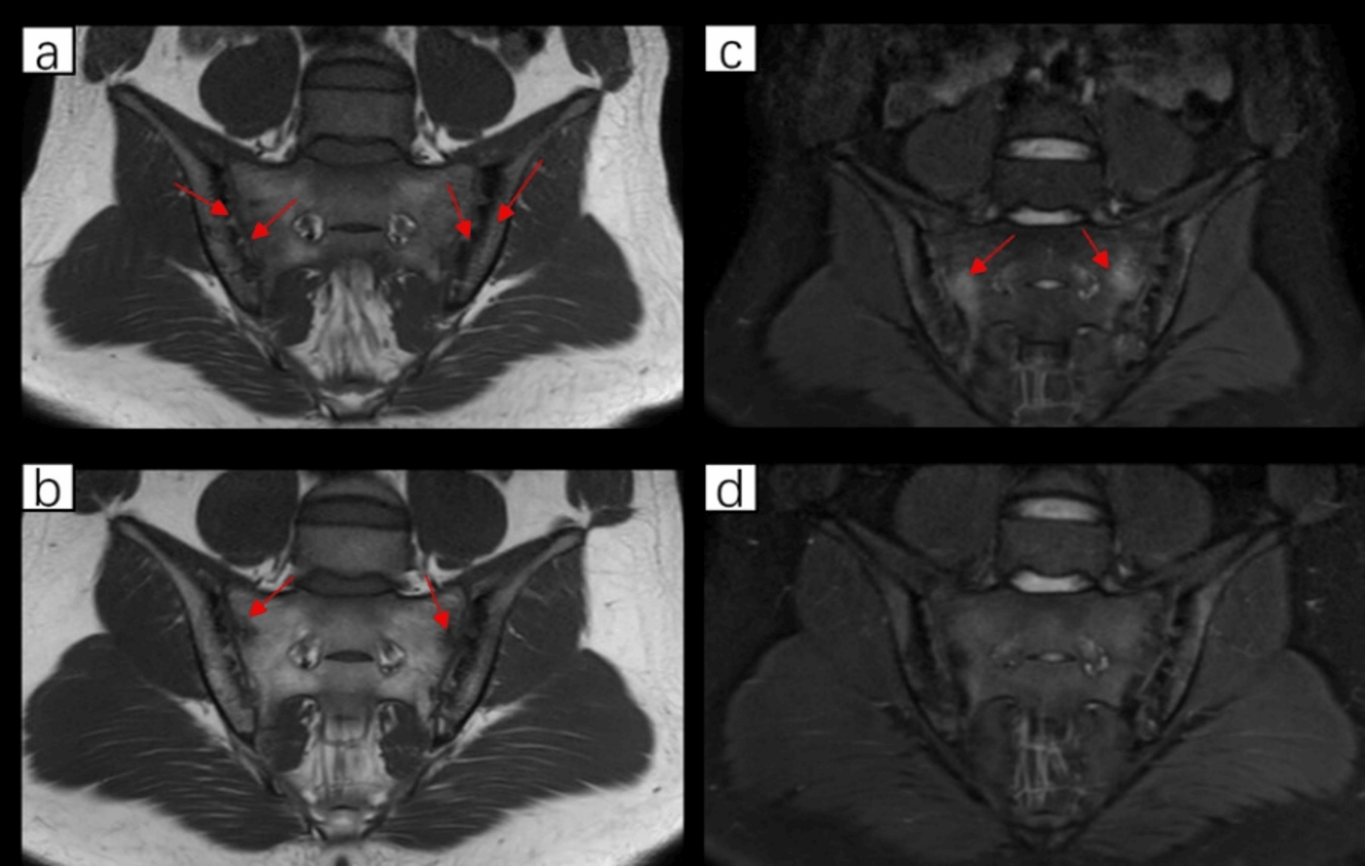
MRI of the sacroiliac joint before and after treatment (a) T1WI, March 2025; bilateral sacroiliac joint surface roughness with multiple worm-eaten-like changes beneath the articular surfaces, accompanied by narrowing of the joint space, is marked with arrows. (b) T1WI, July 2025; the arrow indicates fat metaplasia/depositopn. (c) T2 fat suppression sequence, March 2025; the arrow indicates bone marrow edema. (d) T2 fat suppression sequence, July 2025; the bone marrow edema has disappeared T1WI: T1-weighted imaging
